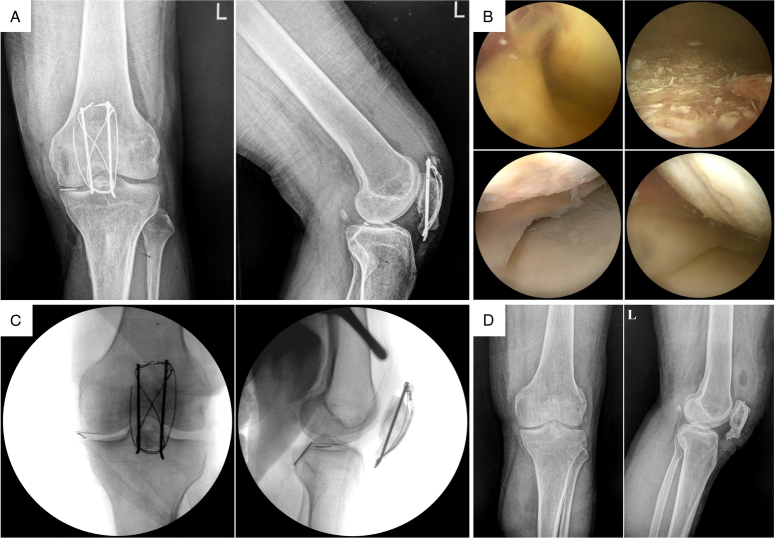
Series of X-ray images of a 43-year-old middle-aged male who underwent open reduction internal fixation surgery for left patellar fracture 7 years ago. He occasionally experienced discomfort postoperatively but did not adhere to regular follow-ups. Recently, he presented with 1-week persistent left knee swelling, pain, and limited mobility. X-ray examination revealed the broken of the patellar tension band wire, which had migrated from the anterior aspect of the patella into the joint space; intraoperatively, arthroscopic findings showed articular cartilage and joint capsule injuries, with no visible wire, but fluoroscopic localization revealed wire migration outside the joint space. The patient underwent arthroscopic and open surgical wire removal. Postoperatively, he received antimicrobial and pain relief medications. Follow-up appointments have been scheduled for ongoing monitoring. (A) Preoperative knee X-ray image showing patellar fracture with heterotopic ossification, multiple broken of the tension band wire, with displaced wire within the joint space. (B) Intraoperative arthroscopic image showing chondral injury on the medial femoral condyle, marked synovial hyperplasia in the suprapatellar pouch, and yellow-colored joint fluid. (C) Intraoperative fluoroscopic image of the knee joint shows that the wire foreign body has moved out of the joint cavity. (D) Postoperative knee X-ray image showing complete removal of the broken wire.
